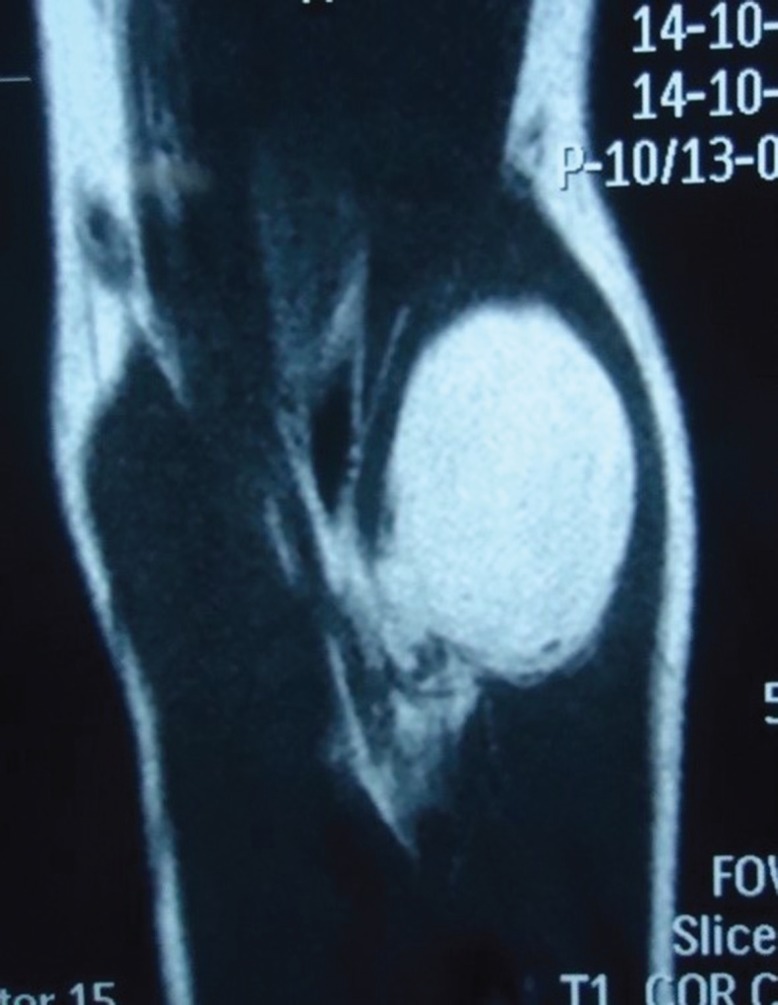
MRI (coronal section) showing relationship of the parosteal lipoma to the underlying radius and the adjacent muscles of the forearm
